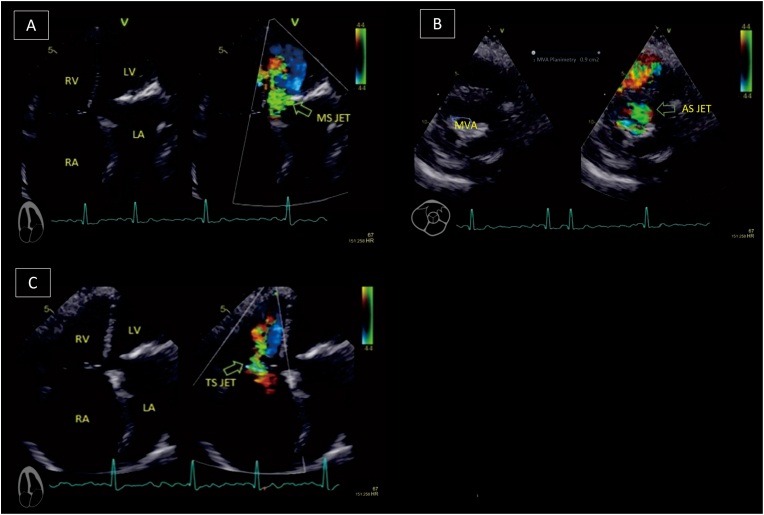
Transthoracal echocardiography showed (A) mitral stenosis in 4 chamber view, (B) The mitral valve area planimetry was 0.9cm^2^ from parasternal short axis view, (F) from 4 chamber view it's seen tricuspid stenosis.
